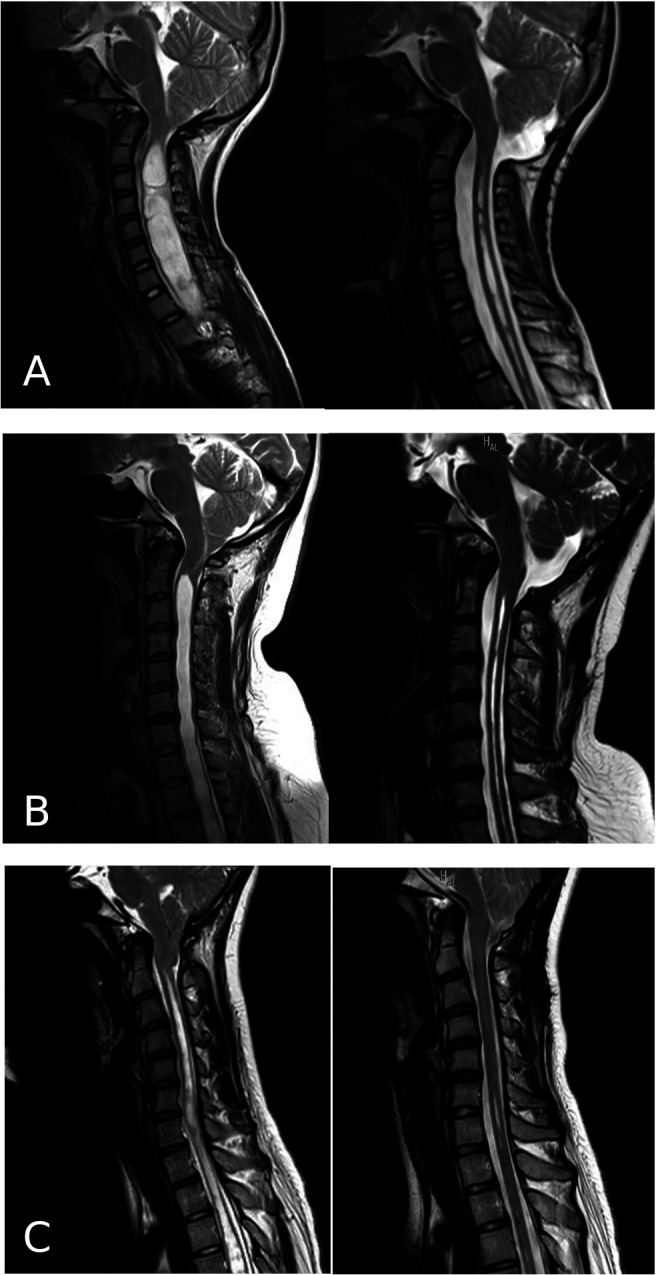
Pre- and postoperative cervical-spine MRI of three representative cases (A, B, and C). The left column shows the preoperative images, and the right column shows the postoperative images.
